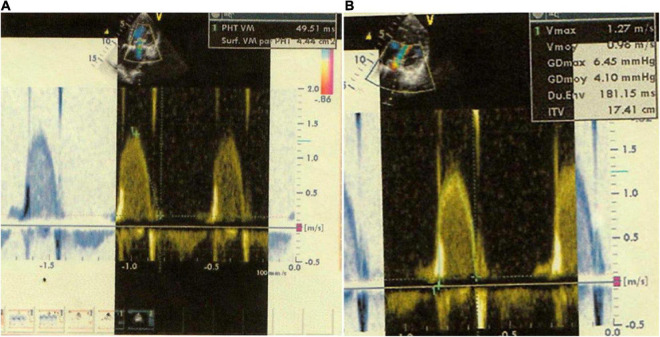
(A) Two-dimensional (2D) apical 4-chamber view shows a mitral valve pressure half-time (PHT) of 49.51 ms. (B) Two-dimensional (2D) apical 4-chamber view shows a transmitral gradient pressure of 4.10 mmHg.
